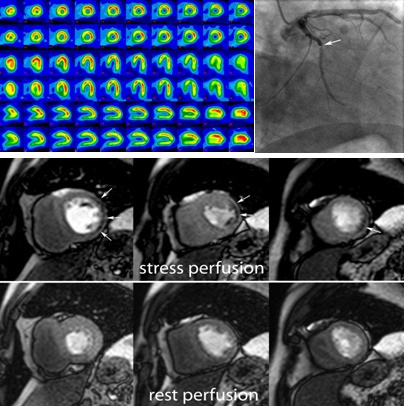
Fig. 5MRI study (rest and stress perfusion) in a 52 year old male with stable angina. Rest and stress (*) SPECT were negative, showing no induced perfusion defects (a). Midventricular short-axis cardiac MRI images (b) of first pass perfusion during rest (bottom) show no perfusion defects while during dipyridamole vasodilatatory stress (top) show a subendocardial perfusion defect in the lateral wall (*arrows*). Coronary angiography (c) confirmed a significant (90%) stenosis of the first lateral branch of Cx (*arrow*, vessel larger than the Cx).
